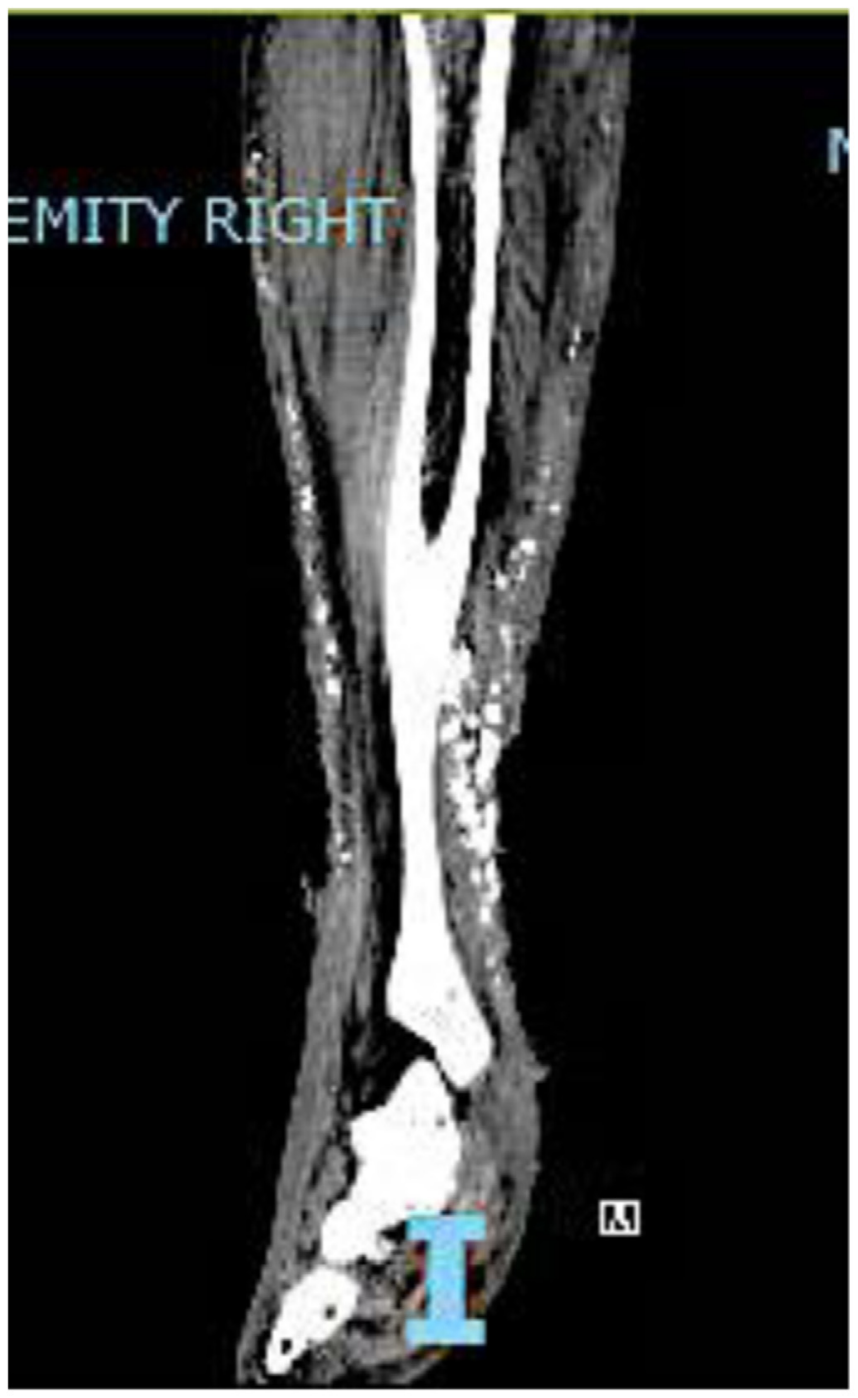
Cross-sectional computed tomography (CT) image showing extensive heterotopic ossification (HO) of the right lower extremity.
